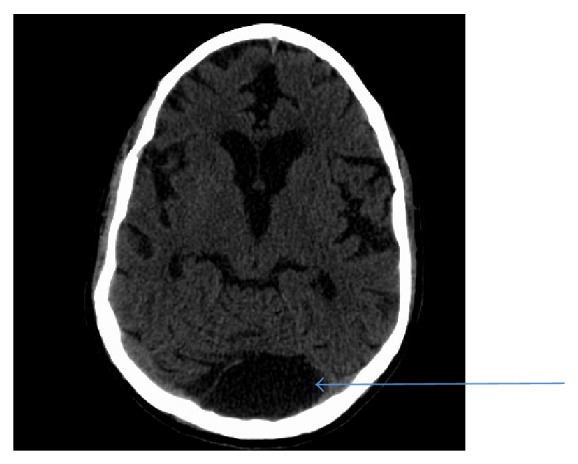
CT of the head without contrast. The ventricles and sulci are prominent, most consistent with global cerebral volume loss. Hypoattenuation of the periventricular white matter is most consistent with chronic small vessel ischemic disease. There is no evidence for intraparenchymal mass lesion or hemorrhage. A large CSF-density space (blue line) is seen posterior to the midline cerebellum, measuring 5.0 × 2.9 cm in image 29 of series 2, most consistent with arachnoid cyst. The gray-white junction is intact. Mild polypoid mucosal thickening is seen within the left maxillary sinus. Mild mucosal thickening is also seen within the right sphenoid sinus. A large right mastoid effusion is seen.
